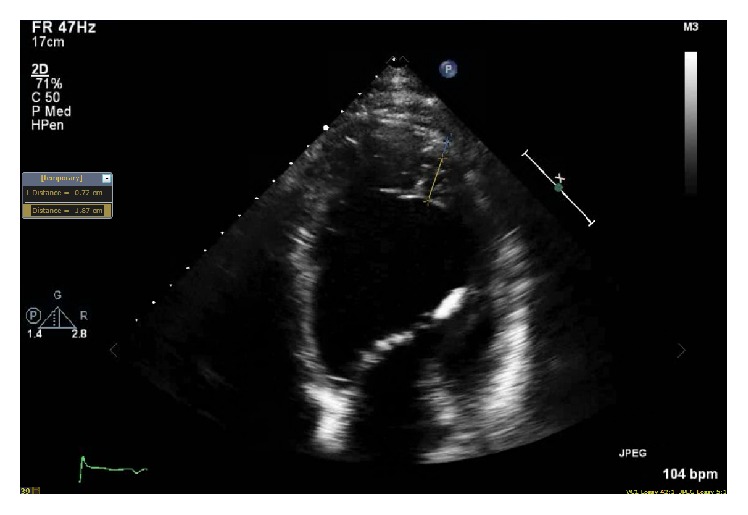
Apical four-chamber view of echocardiography demonstrating the end diastolic ratio of noncompacted layer 18.7 mm (yellow marking) and compacted layer 7.2 mm (blue marking) with resultant ratio of >2.
